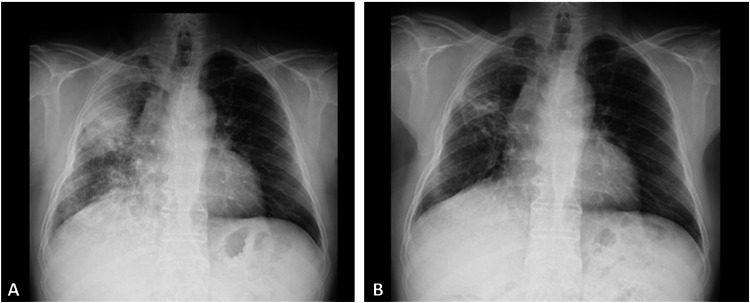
Evolution of the infiltrate in the middle lobe of the right lung on chest radiography (posteroanterior view) A - before treatment; B - after 4 weeks of corticosteroid therapy.
